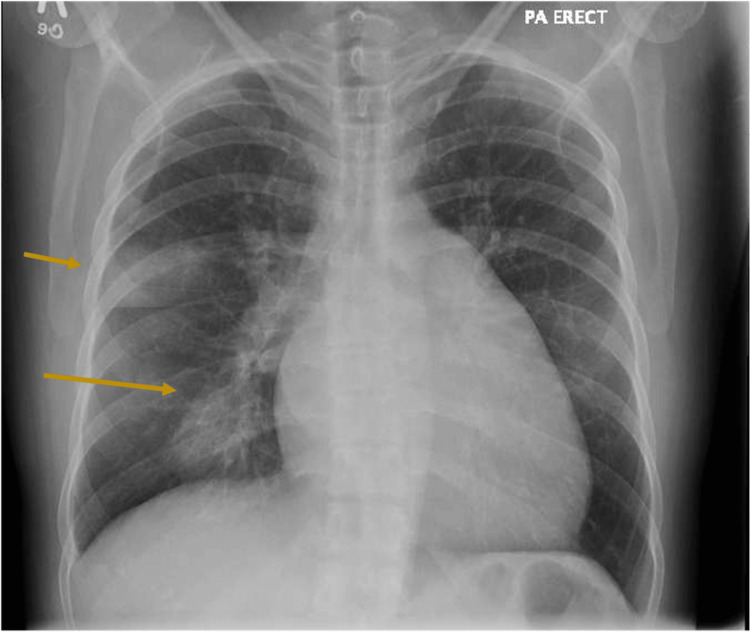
Chest X-ray on the second admission Revealing airspace opacity on the inferior segment of the right upper lobe peripherally and on the right middle lobe.
